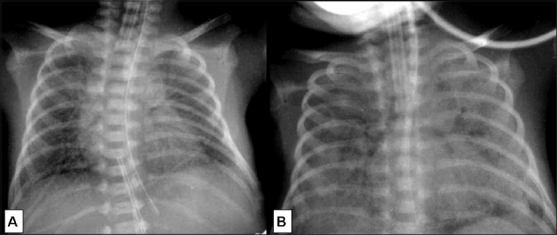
Respiratory distress syndrome (RDS): Portable frontal chest radiograph on day 2 (A) shows reticulogranular opacities throughout both lungs with prominent air bronchograms. The cardiac silhouette is preserved and both lungs are hypoaerated. Frontal radiograph of the chest on day 9 (B) shows more severe changes. There is obliteration of the cardiac and diaphragmatic contours due to marked opacification, giving a complete ‘white out’ appearance
